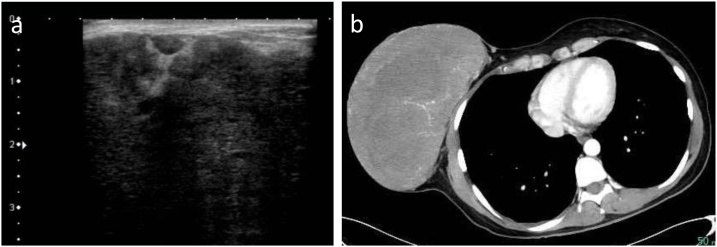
a Ultrasonography shows a circumscribed iso-echoic to hypo-echoic mass replacing the right breast tissue. b Enhanced computed tomography shows a huge tumor, with of 20 cm in diameter with poor internal enhancement.
